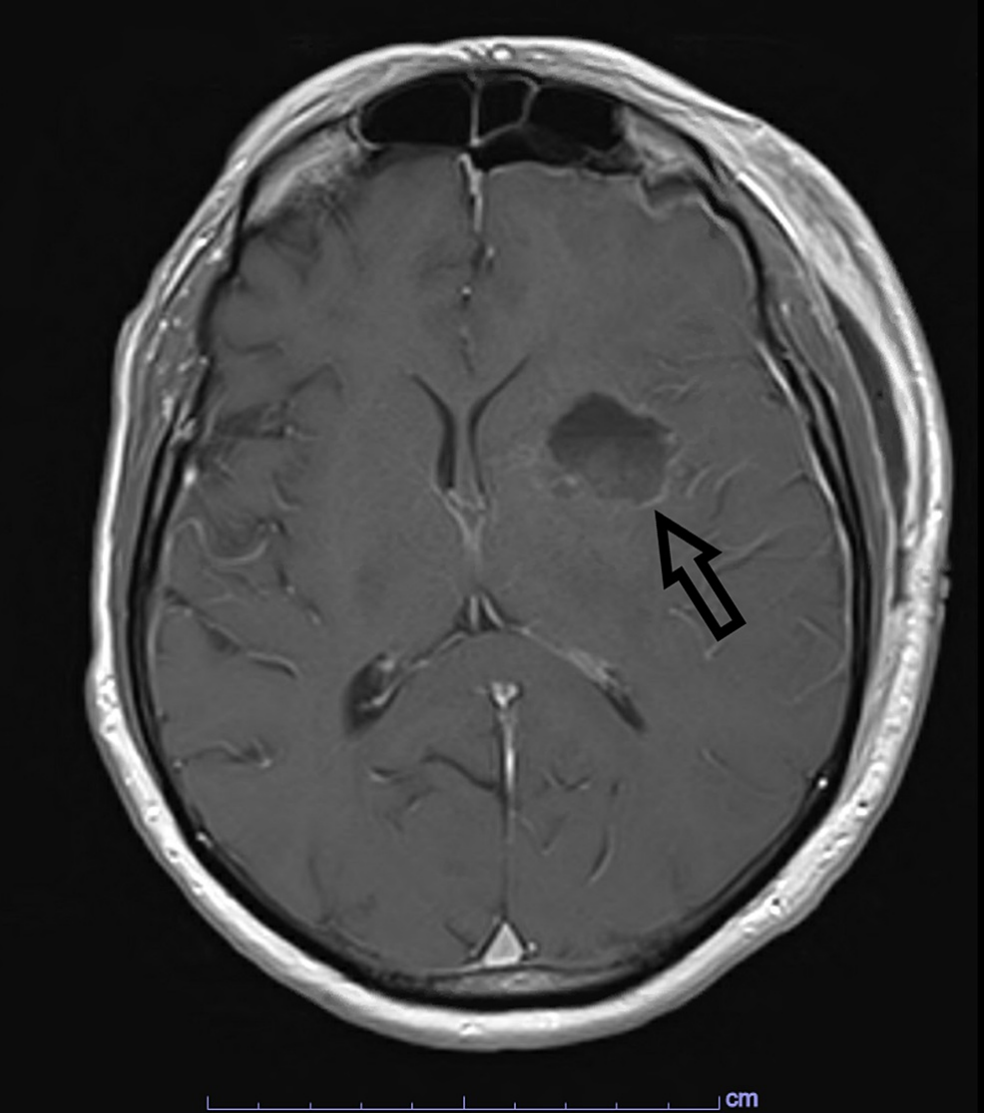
Post-operative, T1-weighted, axial MRI image with contrast demonstrating resected left frontal lesion. Arrow: resection cavity of the left frontal lesion
